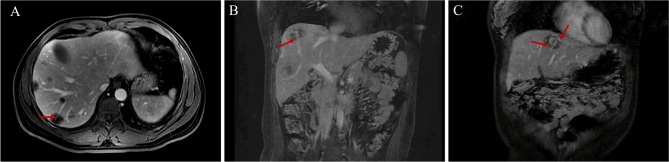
Vascular penetration sign. (A, B) The vascular penetration sign in transverse and coronal MR images. (C) Vascular penetration sign with the portal and hepatic veins in patient 3. The terminal branches of the portal vein are at the bottom, and the branches of the left hepatic vein are at the top.
